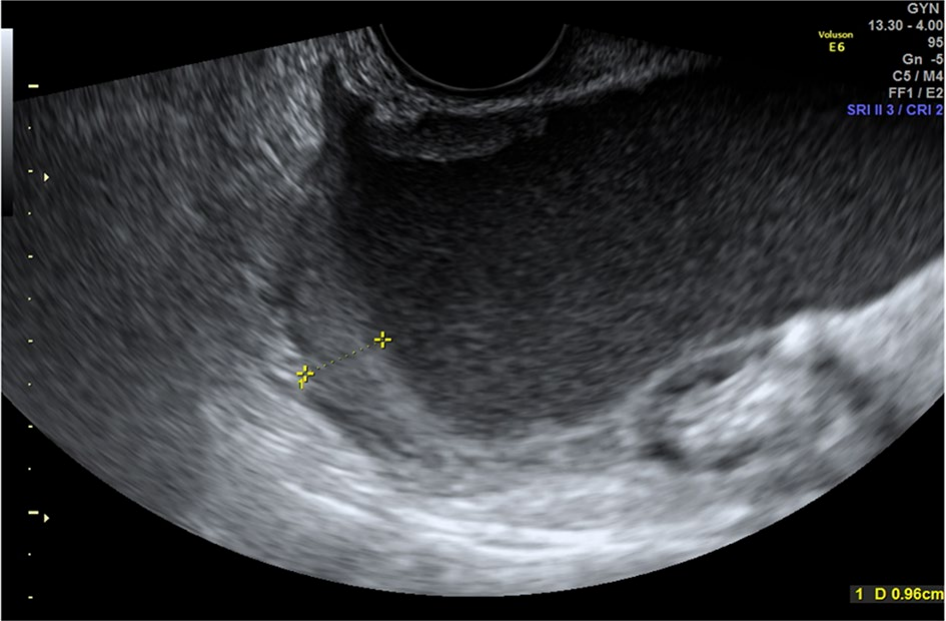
Ultrasound finding of carcinosis. Ultrasound assessment of carcinosis was found to be one of the most important features to predict PFS
